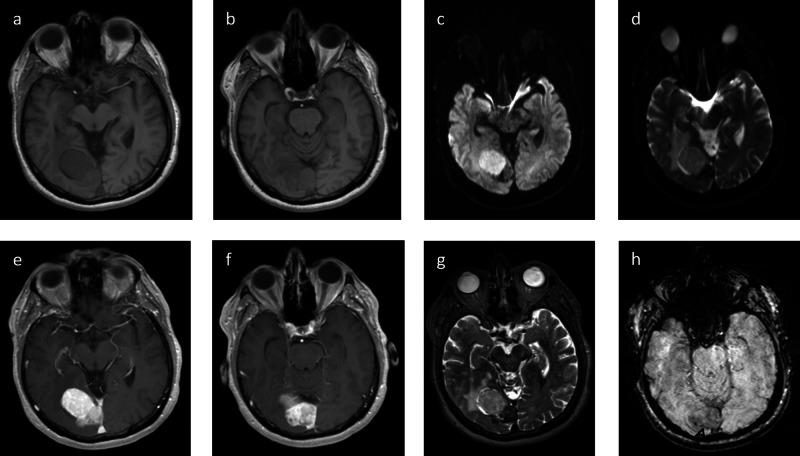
MRI Brain T1-weighted pre-contrast images along the superior (a) and inferior (b) aspects of the mass demonstrate mostly internal isointense signal though the main, somewhat pedunculated component exhibits a slightly T1-weighted hypointense signal relative to the base. Diffusion (c) and ADC (d) demonstrate restricted diffusion involving the main, rounded component. T1-weighted post-contrast images along the superior (e) and inferior (f) aspects of the mass demonstrate avid enhancement, though more homogenous enhancement is seen along the superior rounded component that restricts diffusion. The T2-weighted fat-saturated image demonstrates a hypointense signal along the base of the mass with a mildly hyperintense signal along the superior, rounded component. SWI sequence demonstrates areas of susceptibility artifact (arrowhead) along the base of the mass possibly related to blood products MRI: magnetic resonance imaging; ADC: apparent diffusion coefficient; SWI: susceptibility-weighted imaging
